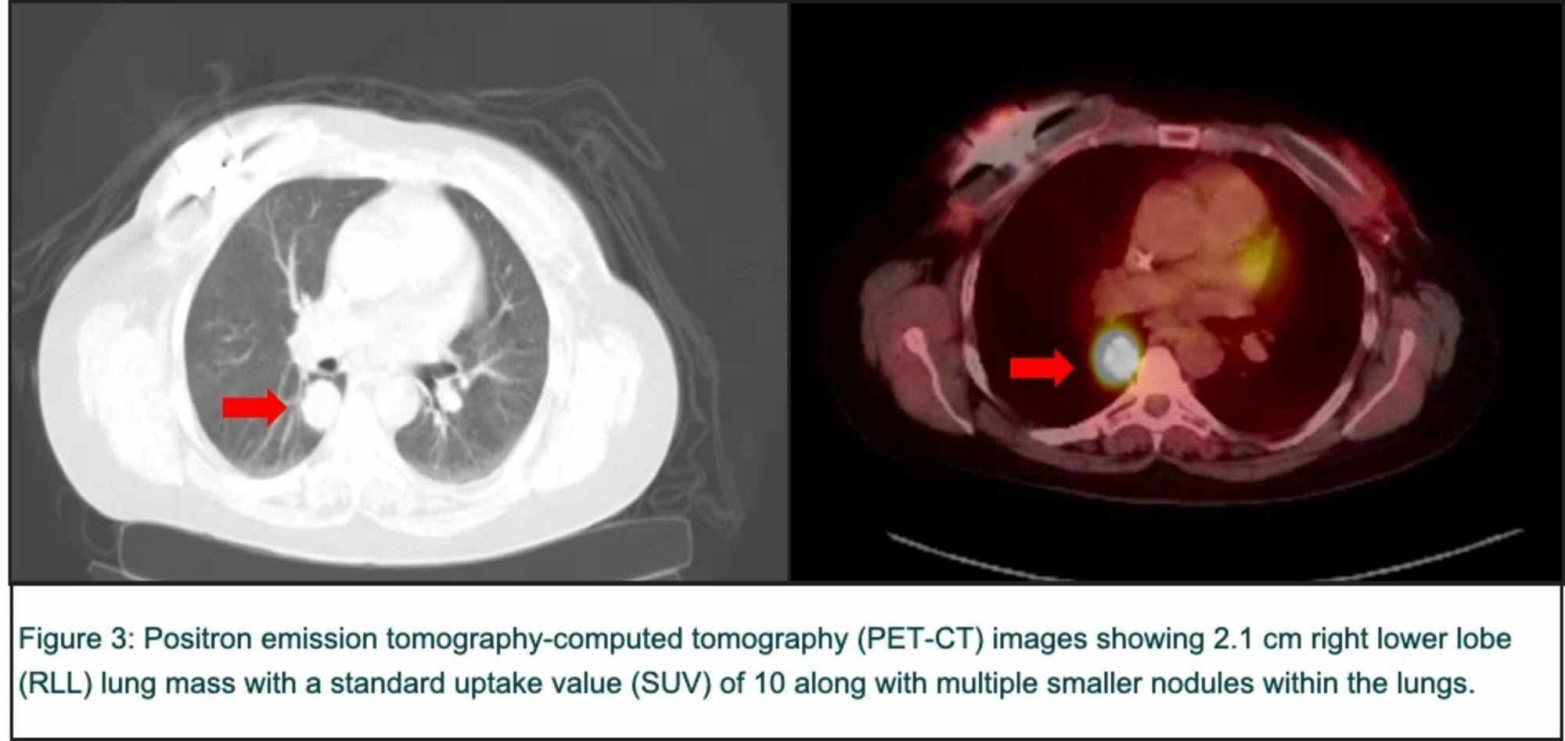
Positron emission tomography-computed tomography (PET-CT) images
